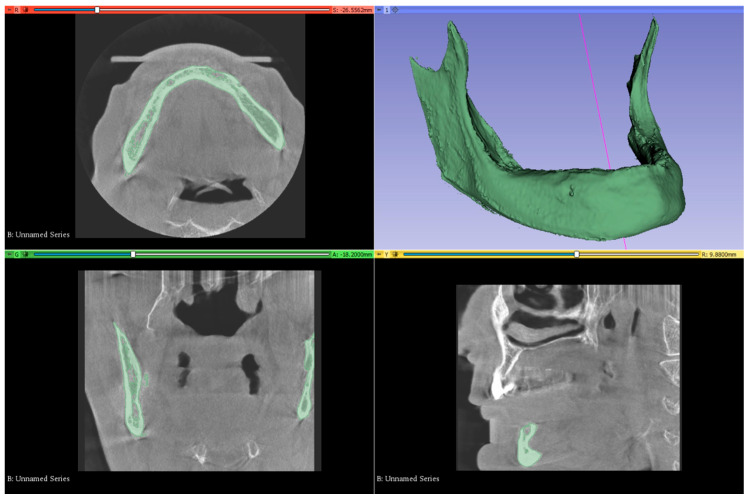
Segmentation of mandibular bone from computed tomography (CT) data and three-dimensional reconstruction using 3DSlicer software.
